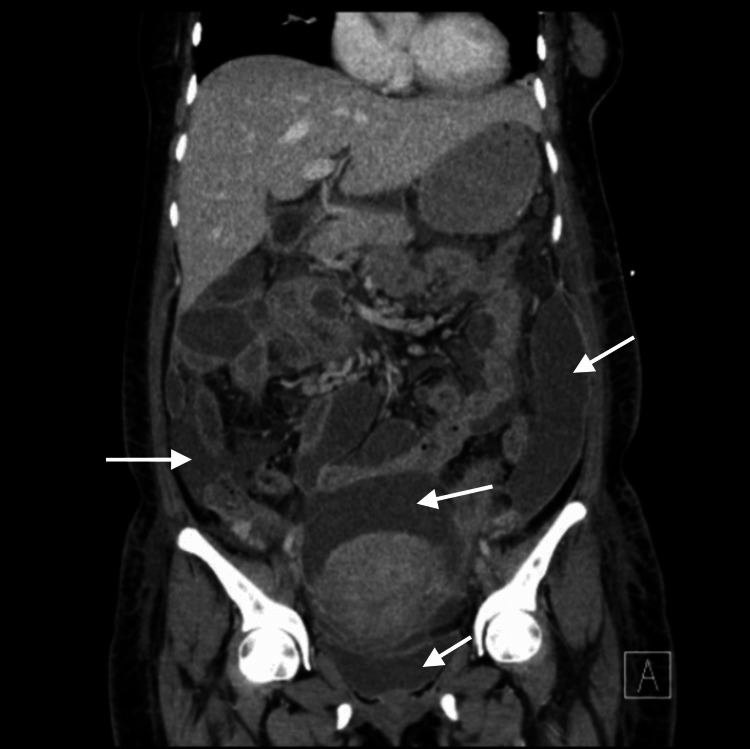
Coronal CT image of the abdomen/pelvis showing multiple fluid collections in the paracolic gutters and surrounding the uterus CT, computed tomography.
